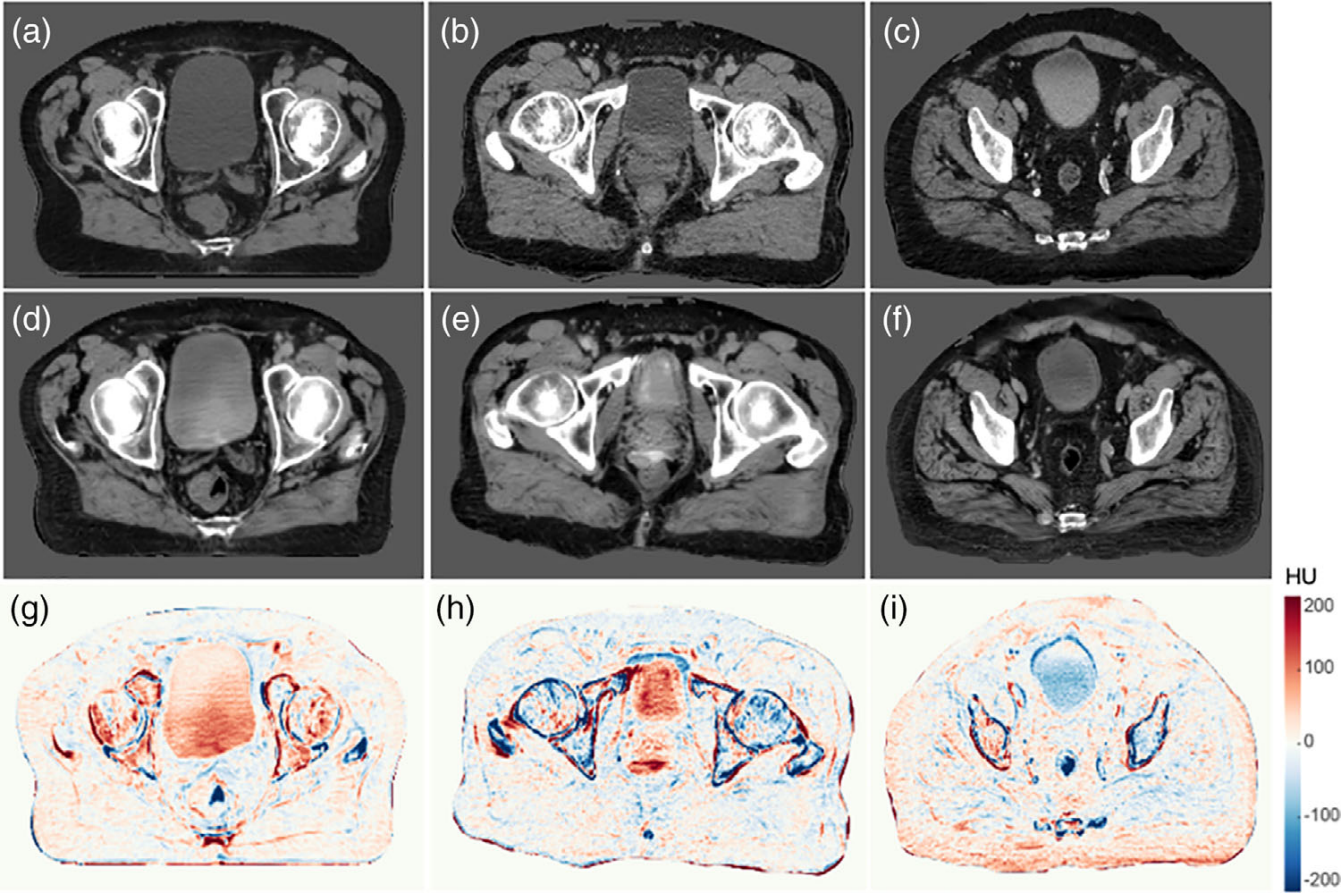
sCT quality assessment. (a–c): Axial view CT images of 3 different patients. (d–f): Corresponding sCT images. (g–i): Difference in HU between CT and sCT.
